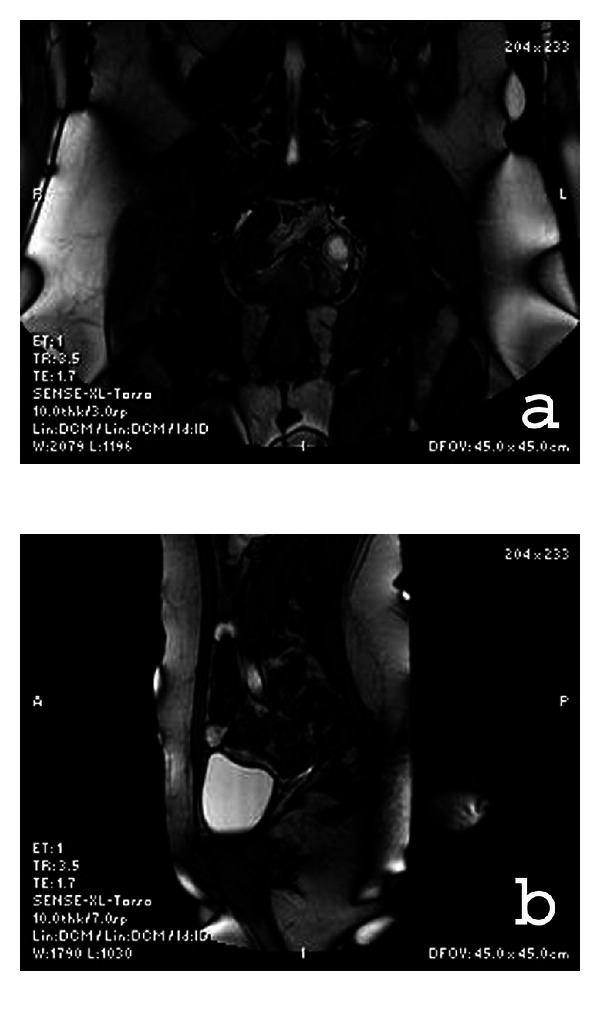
Uro-MRI: (a) and (b) ureteral hyperintense solid bulk below the bifurcation of the left common iliac artery.
